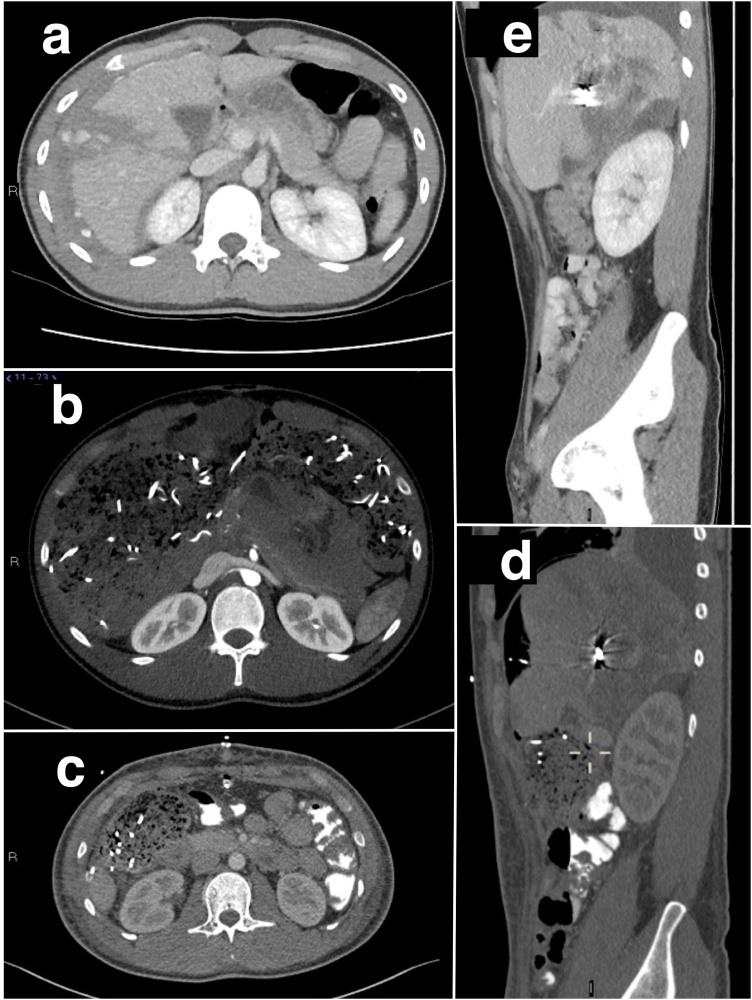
a: Trauma CT of the abdomen demonstrating a complete laceration through the right liver lobe, perihepatic hematoma and ongoing arterial extravasation in the laceration cavity and onwards in the intraperitoneal space. b: Abdominal CT showing the tightly packed abdomen, with visible compression of the retroperitoneal space and drapes directly adjacent to the right kidney. c/d: Abdominal CT showing gauze directly compressing the right kidney indicated by crosshair. e: Abdominal CT approximately ten days post trauma, with visible coils and hepatic lacerations. Right kidney has a normal contour and contrast enhancement.
